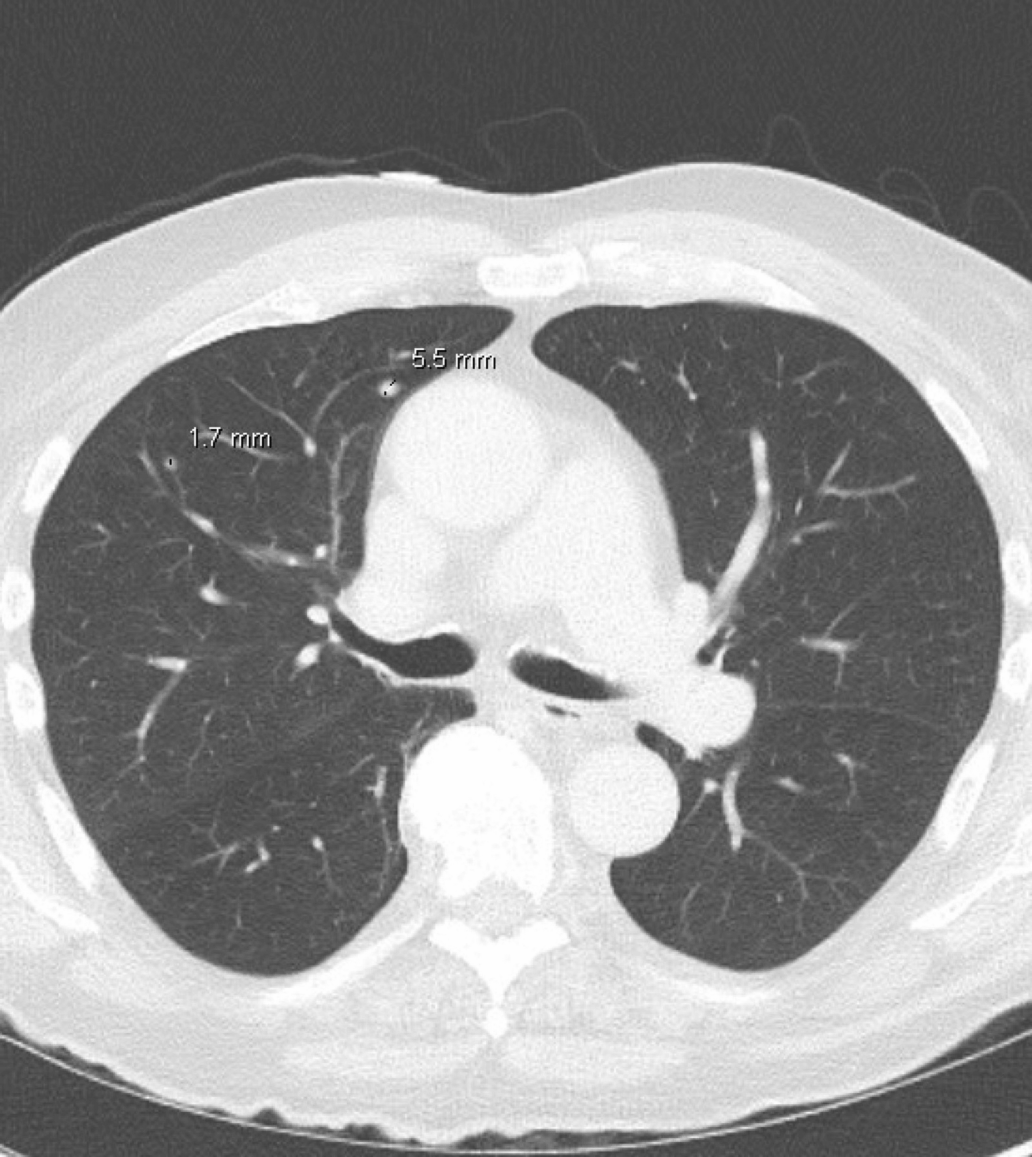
Axial contrast-enhanced CT scan of the chest in lung window demonstrates two non-calcified pulmonary nodules in the right lung measuring 5.5 mm and 1.7 mm.
